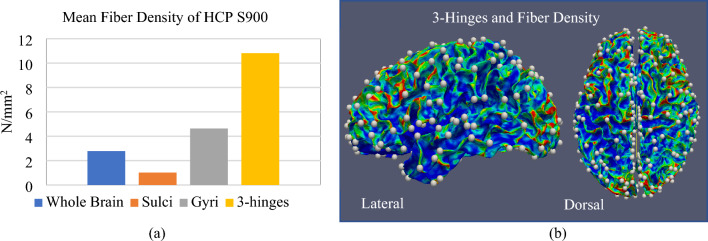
Relation between the density of axonal fibers and morphology of the brain. (a) Mean fiber density of HCP S900^149^ in sulci, gyri, whole brain, and 3-hinge folds. The fiber density in 3-hinge folds is even greater than typical gyri. (b) The density map of an example brain including the detected 3-hinge folds patterns. The locations including 3-hinges folds have greater fiber density than other locations. HCP S900 data includes 900 healthy brain data. The processing and the tractographic reconstruction from the DWI (Diffusion-Weighted Imaging) data were carried out using functions from the MRtrix3 software package^150^. For each subject, 5 × 10^4^ fiber tracts were reconstructed. The fiber density of all individuals in the HCP S900 dataset was calculated and then the mean fiber densities of the whole brain, sulci, gyri, and 3-hinges were counted.
